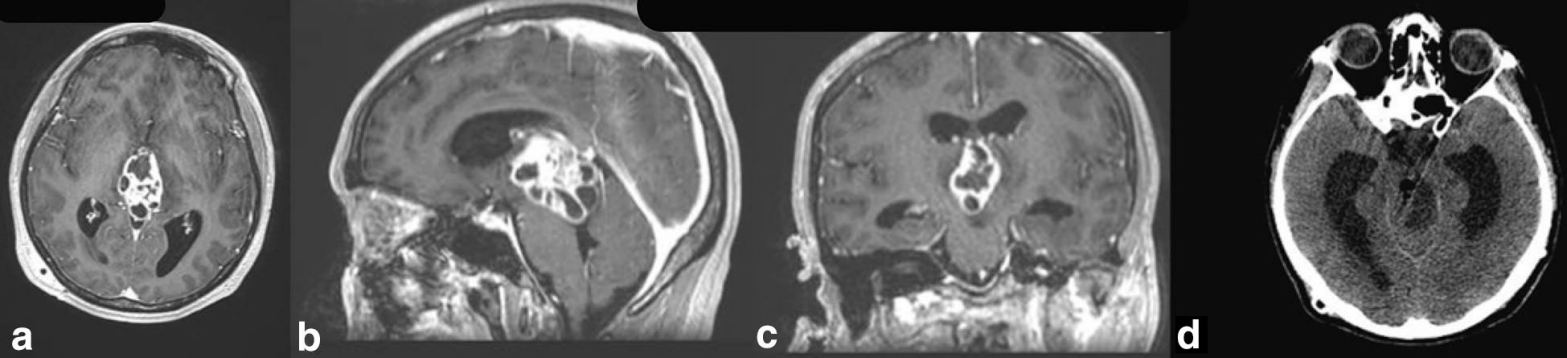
A multi-cystic heterogeneously enhanced mass is observed in the third ventricle on T1-weighted brain MR (a–c). Temporal horns are dilated despite previous V-P shunt surgery (d)
